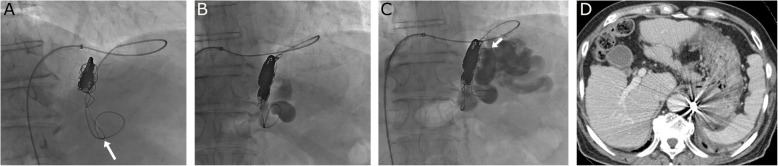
a The steerable catheter was inverted from distal to the coil embolization site (white arrow). Detachable coils were deployed using a 1.9 Fr micro catheter through the inverted steerable catheter. b Complete coil occlusion of the outflow shunt was confirmed and the inferior phrenic vein was not visualized. c We gradually injected 5% ethanolamine oleate with iopamidol (total 24 ml) through the microcatheter (white arrow) to embolize up to the coil in outflow shunt, varices, other minor collaterals. d Contrast enhanced computed tomography performed 1 week after coil-assisted retrograde transvenous obliteration revealed that complete occlusion of the gastric varices (white arrow) was achieved (portal venous phase)
